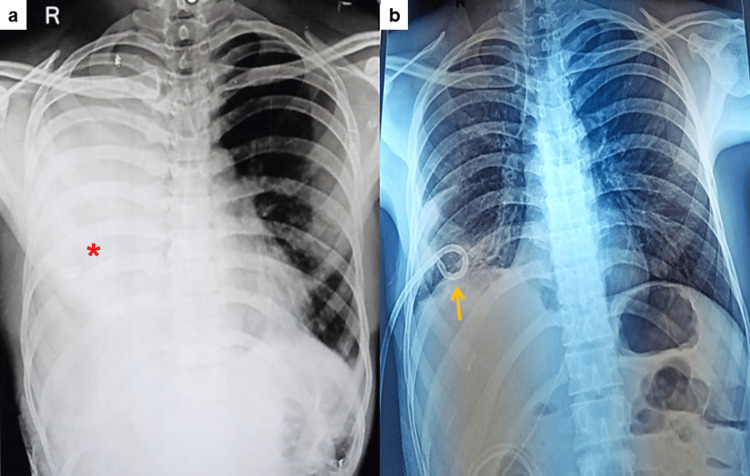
Post-PCNL chest X-ray (POD 3rd) showing (a) massive hydrothorax (highlighted by *) and (b) management with intercostal drainage (highlighted by arrow). PCNL, percutaneous nephrolithotomy; POD, postoperative day
